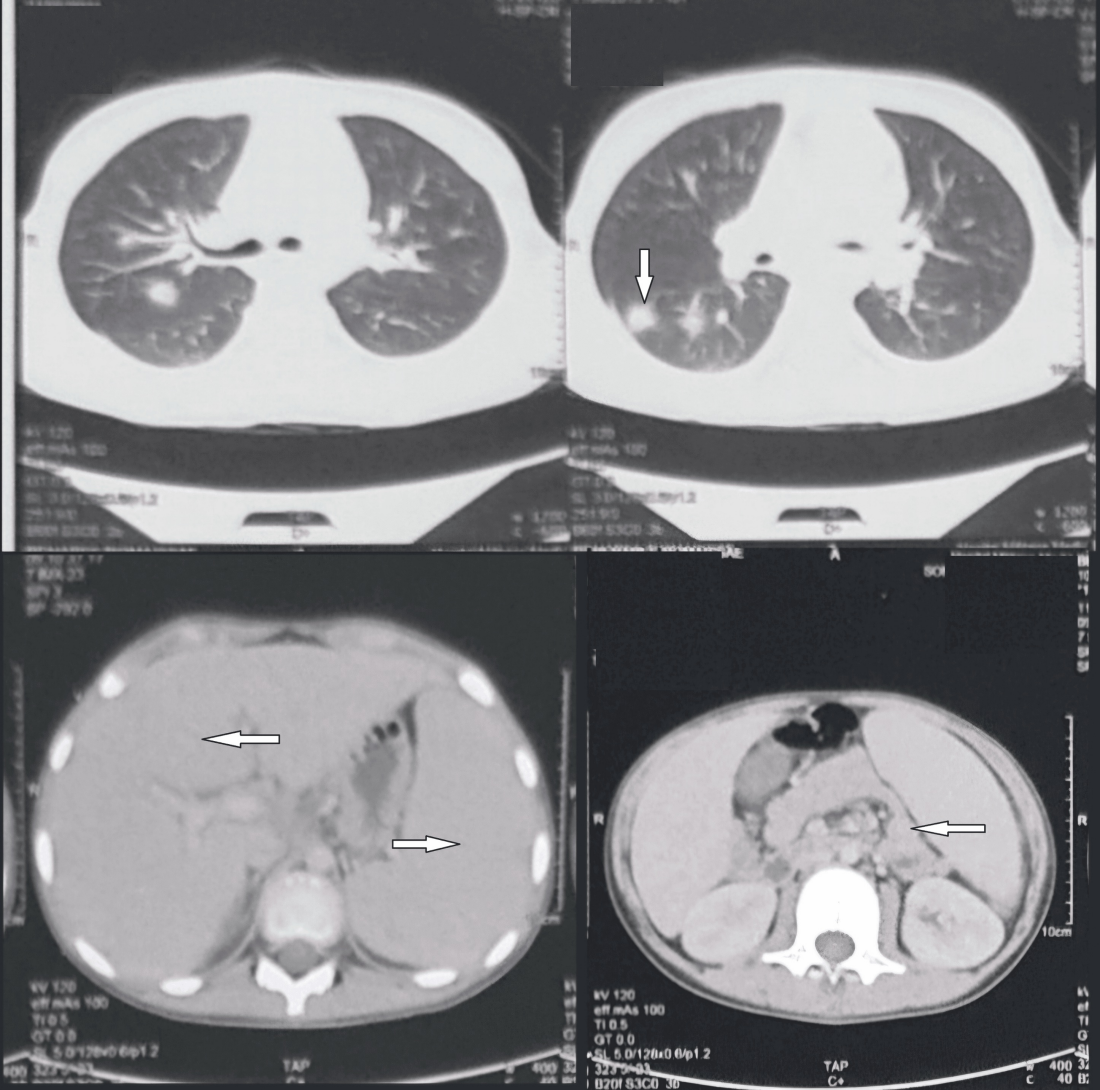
Images from the cervical and thoraco-abdomino-pelvic CT scan of the first patient showing homogeneous hepatosplenomegaly with supra and subdiaphragmatic adenopathies and bilateral mixed pulmonary nodules.
